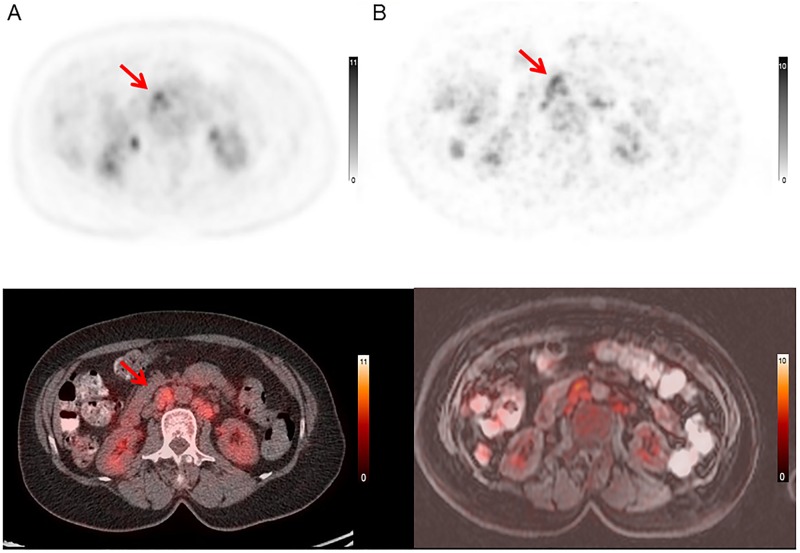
PET/MRI negative, PET/CT positive case. 69 year-old female with malignant lymphoma who underwent PET/CT for initial staging. (A) PET/CT image (upper: PET image from PET/CT, lower: fused PET and CT) (B) PET/MRI image (upper: PET image from PET/MRI, lower: fused PET and MRI). PET/CT could identify the para-aortic lymph nodes. In PET/MRI, PET/MRI could not distinguish these lesions from physiological FDG uptake in the small intestine, even in retrospect.
